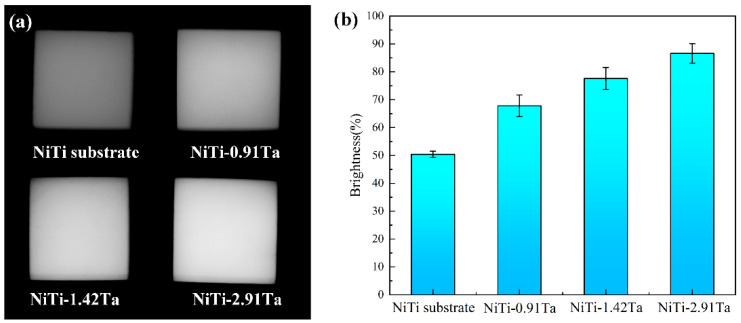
(a) X-ray images and (b) brightness of the NiTi substrate and NiTiTa coatings with different Ta contents.
